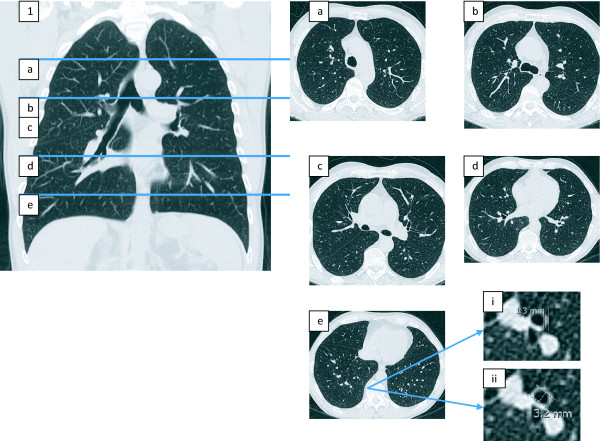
Coronal reconstruction from a non-contrast high resolution computed tomogram (CT) of the thorax with axial sections marked (a) at the level of the top of the aortic arch, (b) at the carina, (c) 1 cm below the carina, (d) at the level of the midpoint of the right pulmonary vein, and (e) 2 cm above the dome of the right hemi-diaphragm. The corresponding axial slices are shown (a-e). Representative measurements of airway wall (W) and diameter (D) are shown, taken from slice (e), (i) and (ii) respectively.
